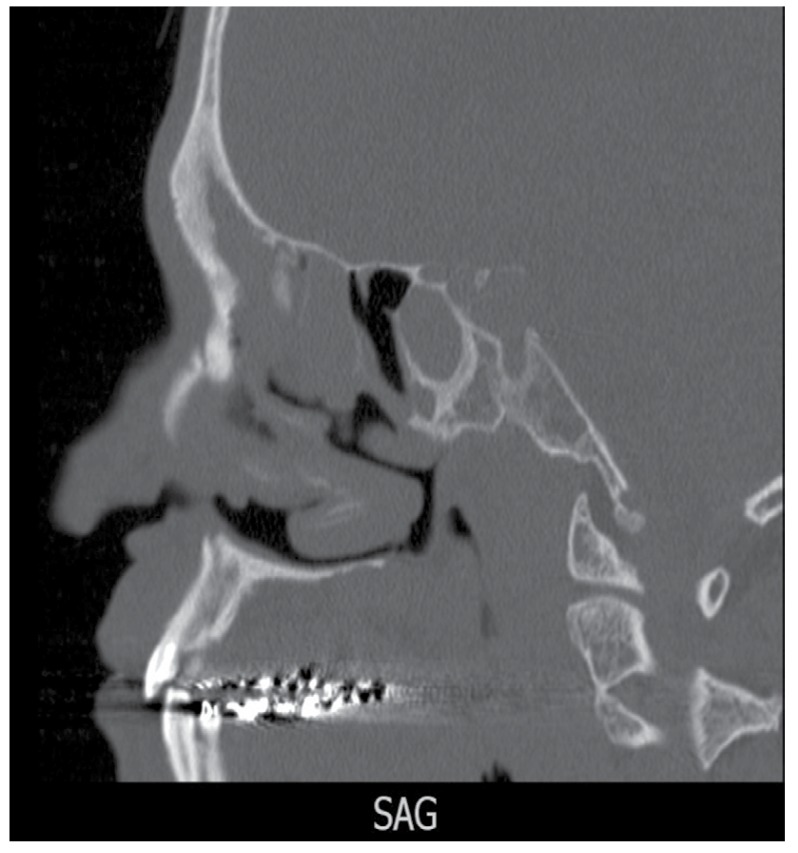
Sagittal CT scan of the sinuses showing frontal sinus aplasia in a 40-year-old patient with cystic fibrosis.
